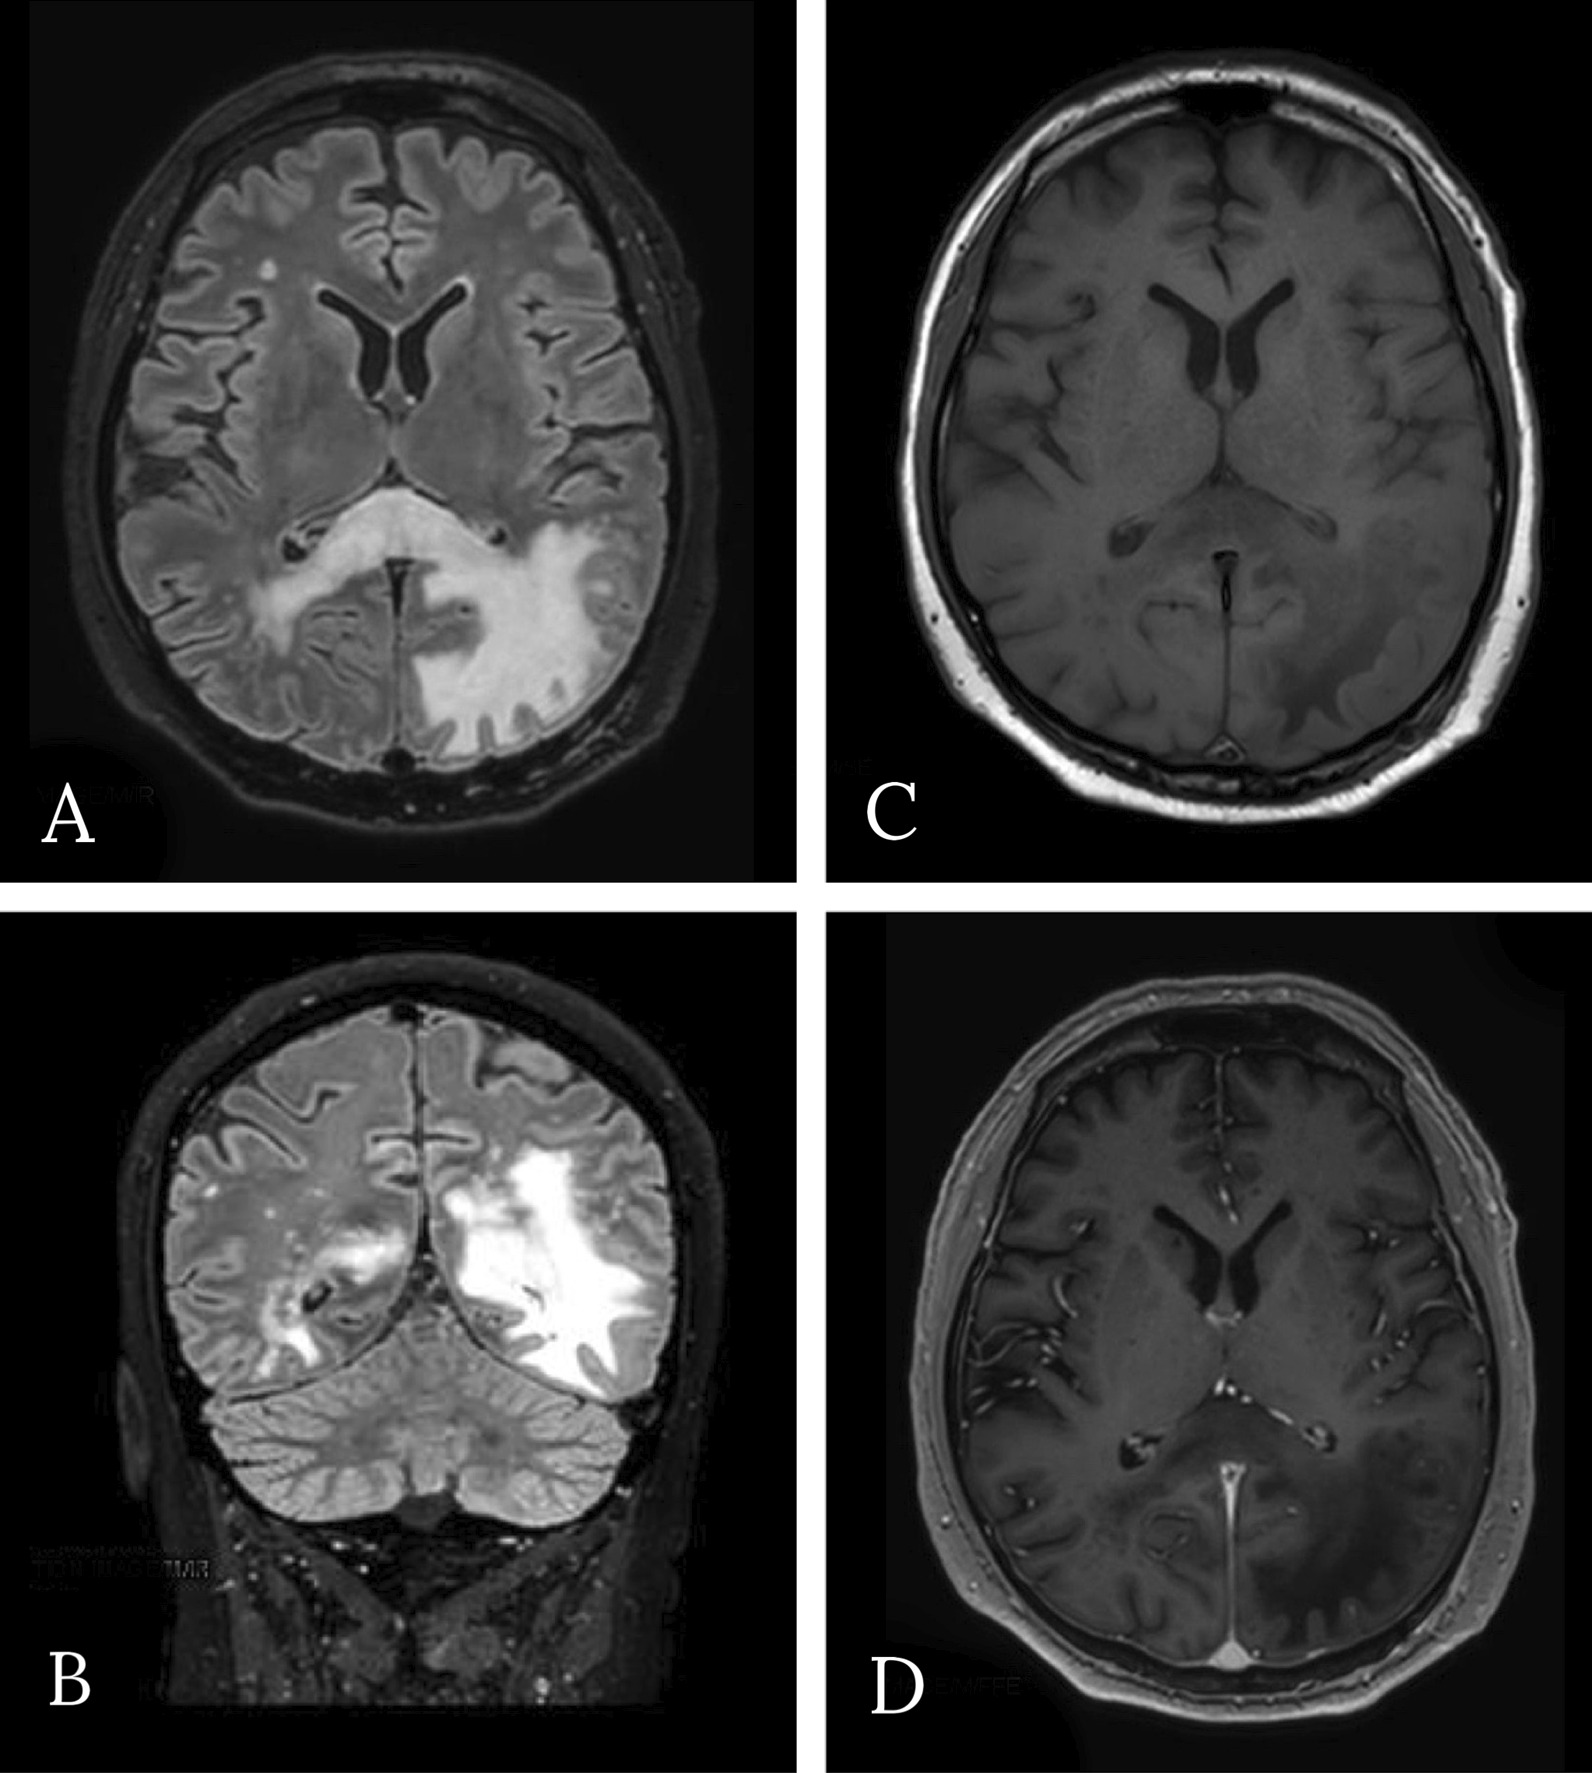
A, B Axial and coronal fluid-attenuated inversion recovery; C T1-weighted; D after administration of gadolinium
